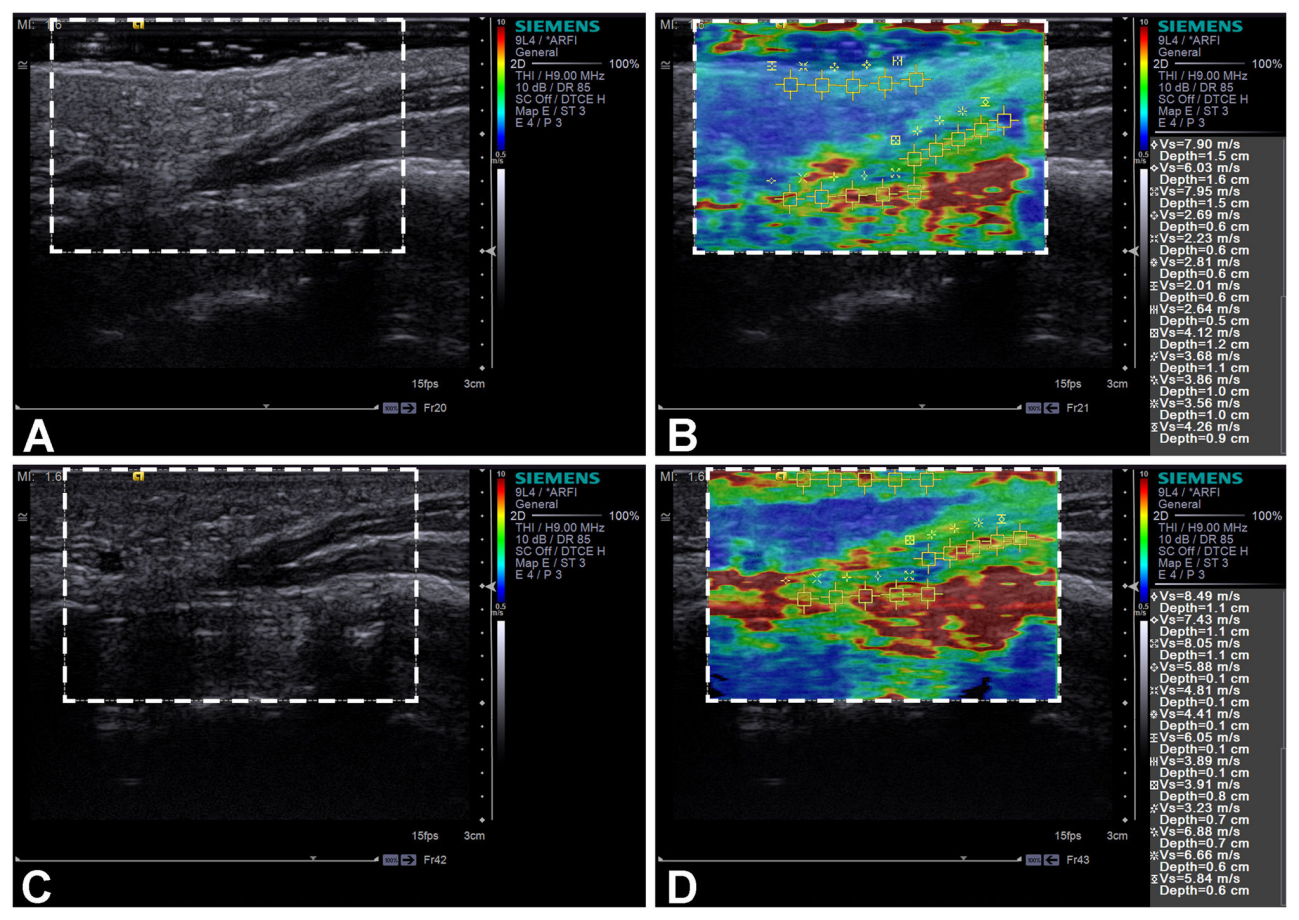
Representative B-mode and ARFI ultrasound images of the transverse carpal ligament. For the session without compression, a thick ultrasound gel layer is visible in both the B-mode (A) and the ARFI (B) ultrasound images. For the session with compression, the skin fold on the palm is flattened and the gel layer is visually absent in both the B-mode (C) and the ARFI (D) ultrasound images. SWV color maps of 30 mm (width) × 20 mm (depth) are shown in (B) and (D). The display scale of the color map is 0.5-10 m/s. The color correlates with tissue stiffness. In general, red, green, and blue areas correspond to high, medium, and low stiffness regions, respectively. Fifteen ROIs were placed on each of the SWV color map to obtain the shear wave velocity measurements.
